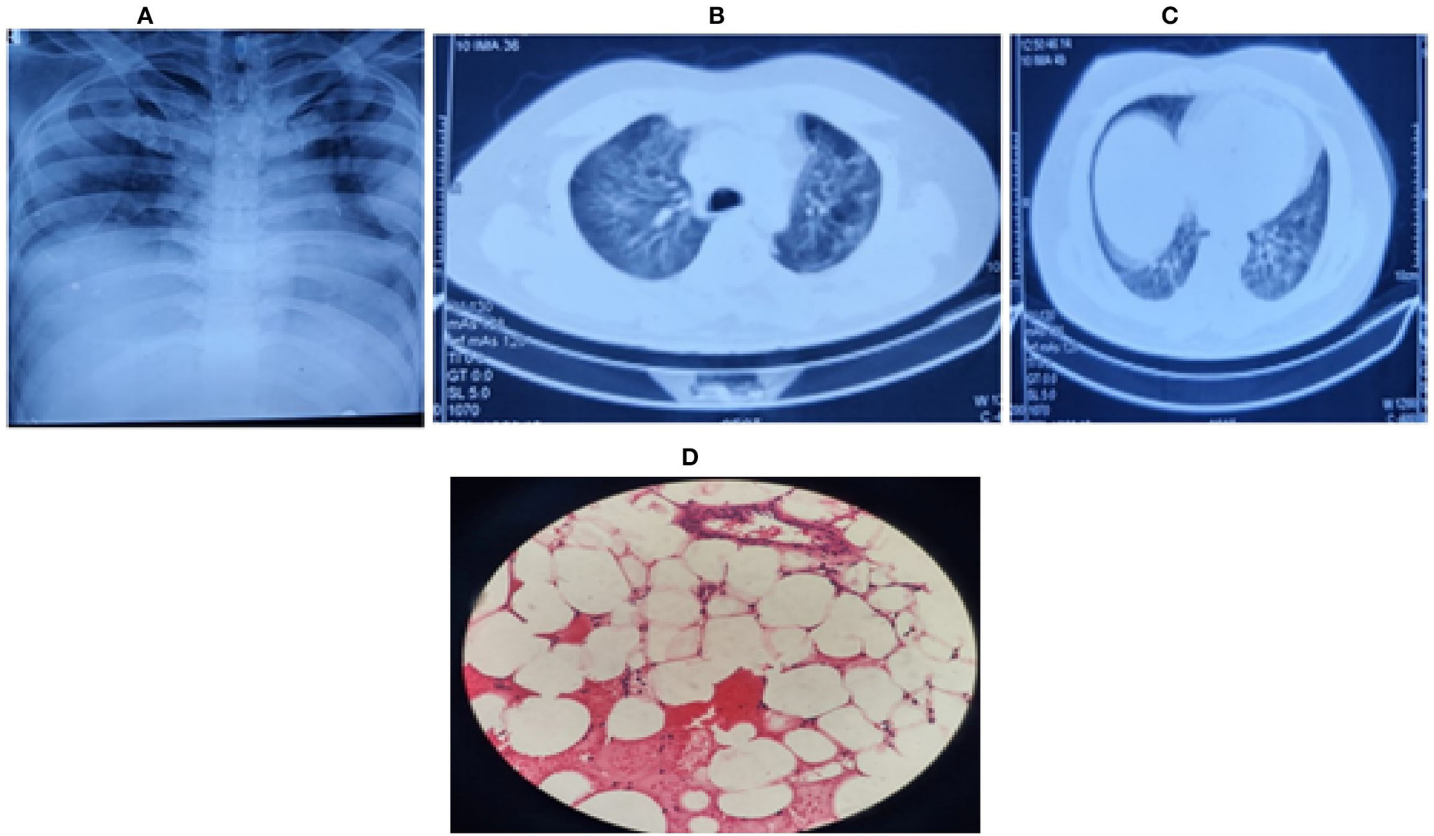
(A) Chest radiograph (bedside) on first day, showing bilateral middle zone haziness, (B) Contrast enhanced CT (CECT) chest on second day showing bilateral ground-glass opacities in lung parenchyma with (C) left upper lobe consolidation and air bronchogram. (D) Bone marrow biopsy showing hypocellular marrow (overall cellularity < 15%), lacunar spaces extensively replaced by fat cells; the residual cellularity mostly included lymphocytes, plasma cells, mast cells, and macrophages with only a few hematopoietic cells.
